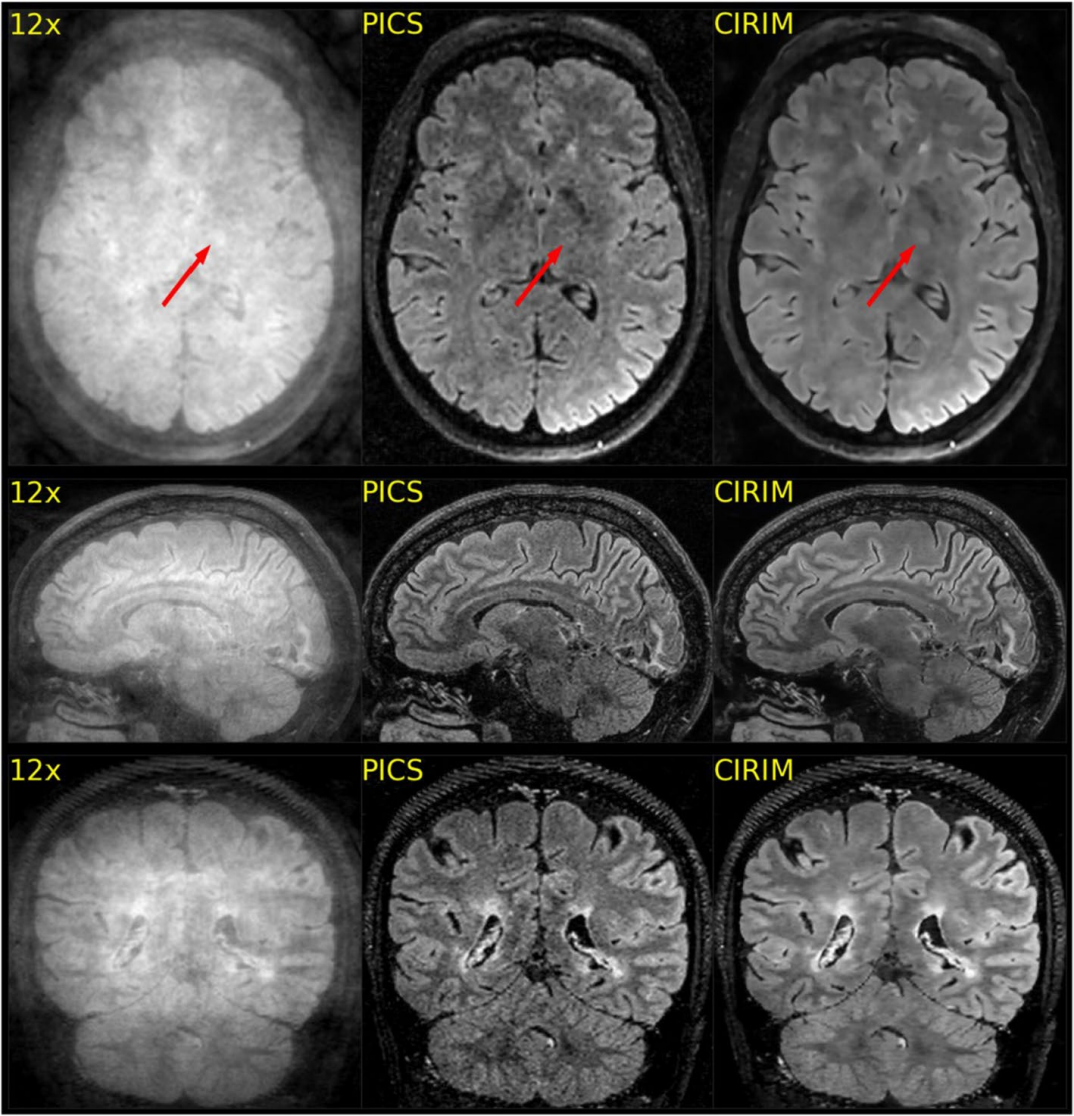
Reconstructions of 12 times accelerated FLAIR scans for three different subjects, where the CIRIM was able to generate better image quality. In the top row, PICS could not accurately depict the left internal capsule lesion (example indicated by the arrow), whereas the CIRIM preserved the contrast
